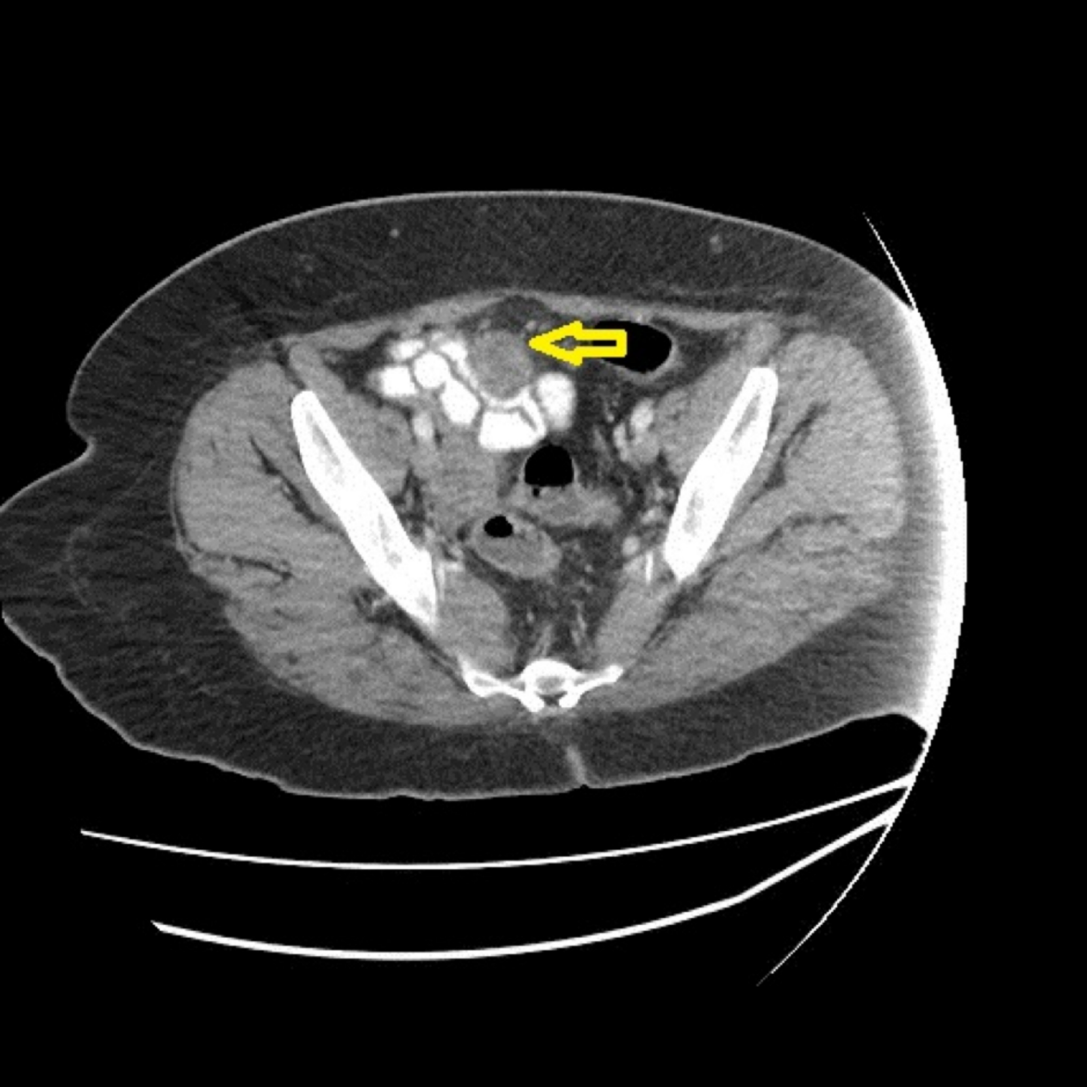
Initial CT of the abdomen and pelvis - coronal view The image shows an extensive irregular wall with luminal narrowing involving the terminal ileum with an eccentric mass as depicted by the yellow arrow CT: computed tomography
